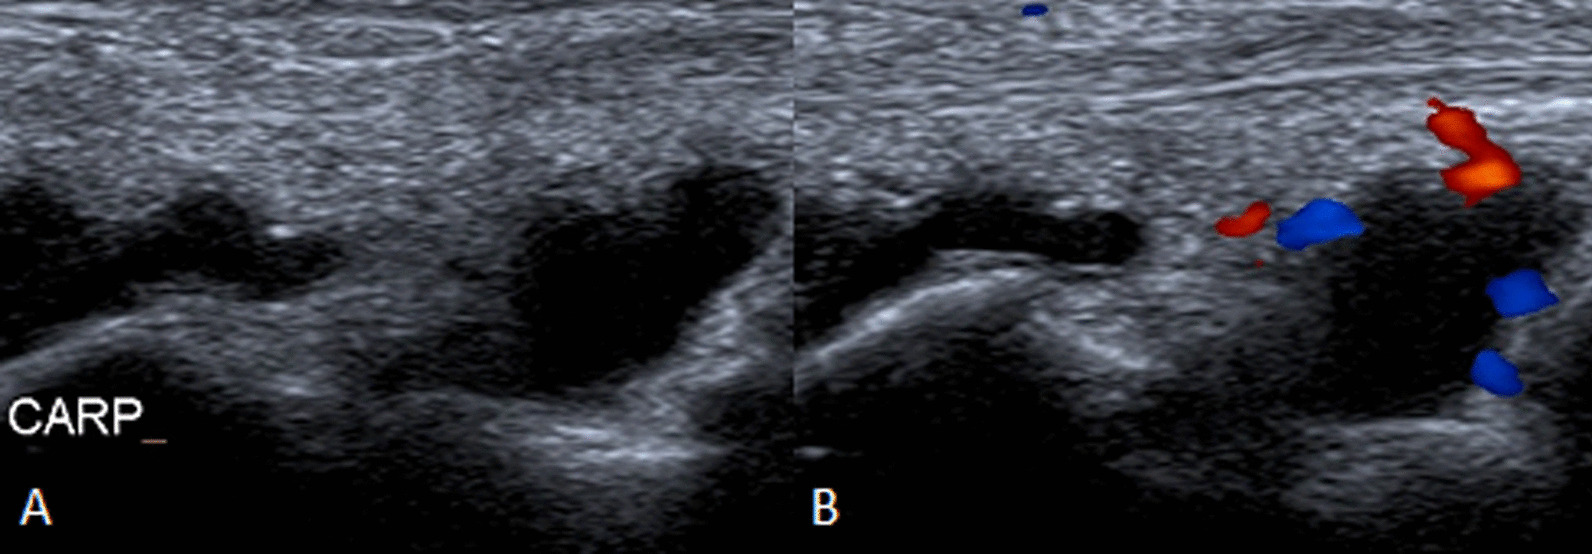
Ultrasound of the wrist (A, B), with positive Doppler signal (B)
